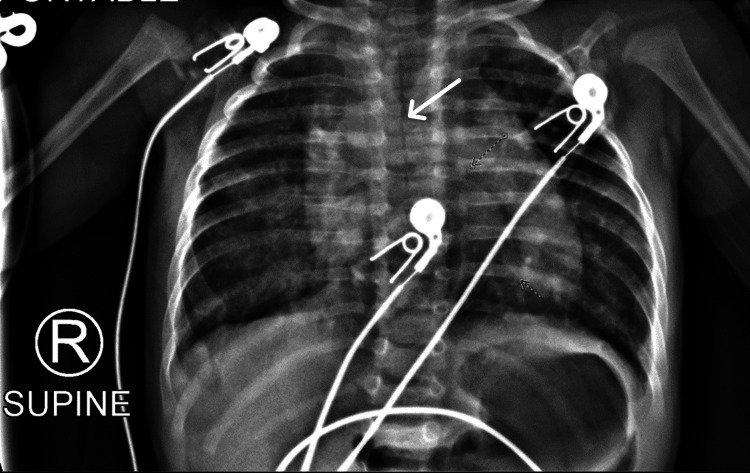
Chest radiograph. The chest radiograph before intubation is consistent with the acute respiratory viral infection. Additionally, there is a significant narrowing of the trachea noted.
